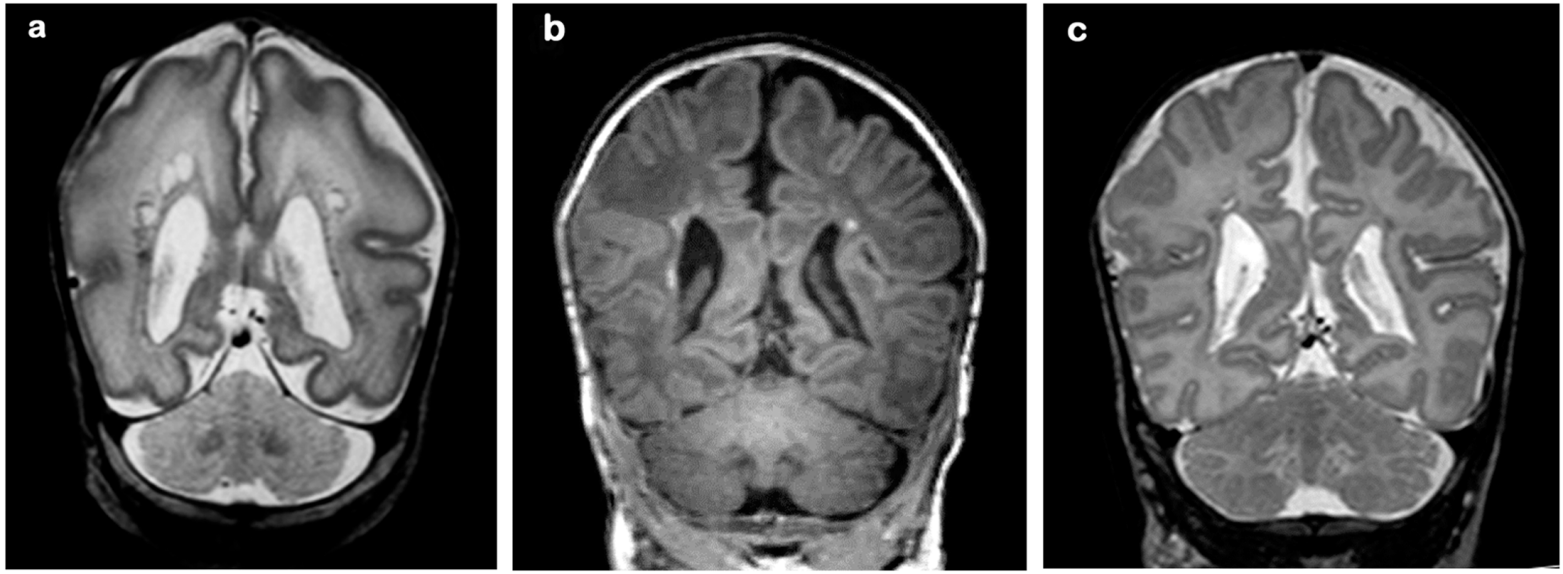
Same infant as above. (a) Early coronal T2-weighted MRI (day 27) showing extensive cysts, more prominent on the right. (b) The cysts are no longer seen on the T1-weighted TEA-MRI but extensive high signal intensity changes and white matter loss are seen instead, more marked on the right side; and (c) a single small cyst on the right is still seen shown on the T2-weighted MRI.
